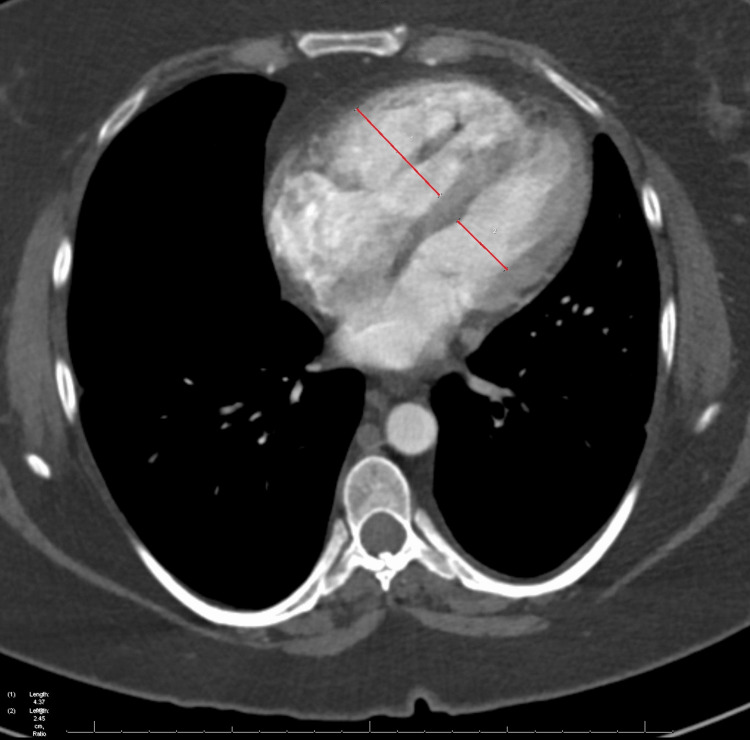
A CTA of the chest in axial view demonstrates right heart strain with an enlarged RV in comparison with the LV. The RV/LV ratio was measured to be 1.6. CTA: computed tomography angiography; RV: right ventricle; LV: left ventricle
